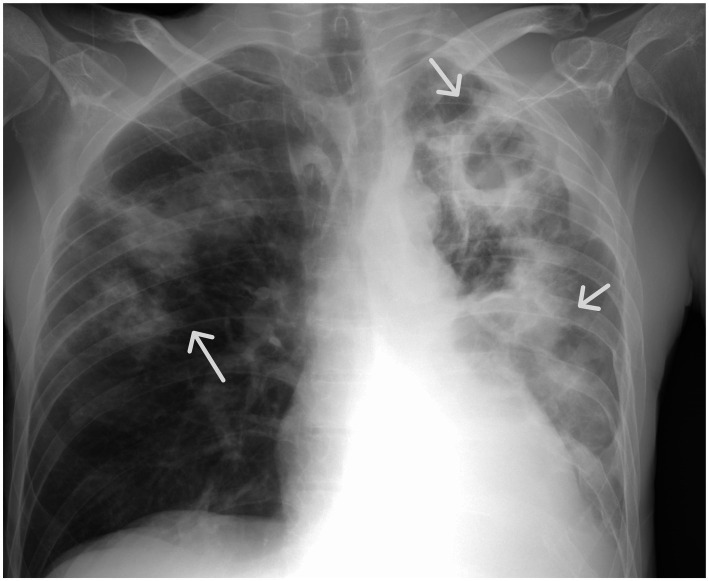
Chest X-ray (anterior–posterior position): Bilateral excavated lung lesions.
